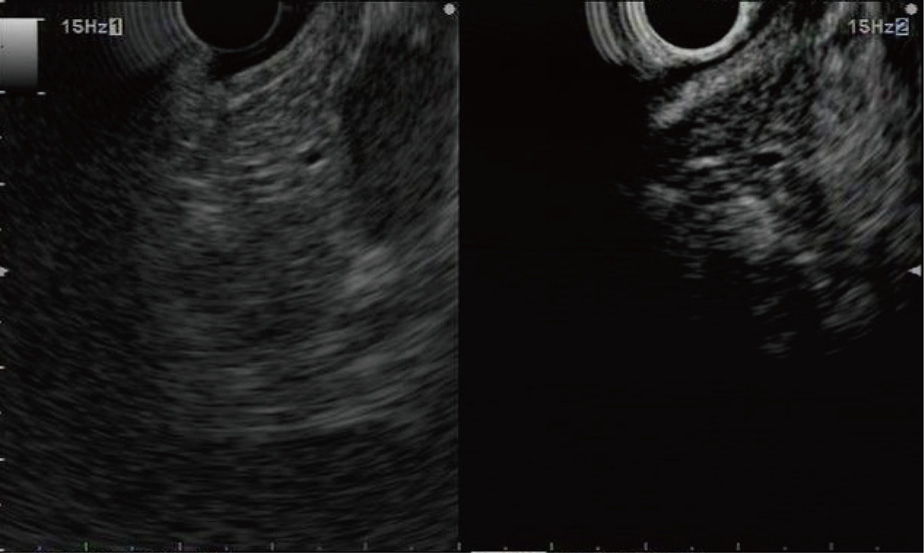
Representative example of a pancreatic neuroendocrine tumor with hyperenhancement. Conventional endoscopic ultrasonography (left) shows a hypoechoic mass with a clear margin at the pancreas body. Contrast-enhanced harmonic endoscopic ultrasonography (right) indicates that the mass has a hyperenhancement compared to the surrounding tissue.
